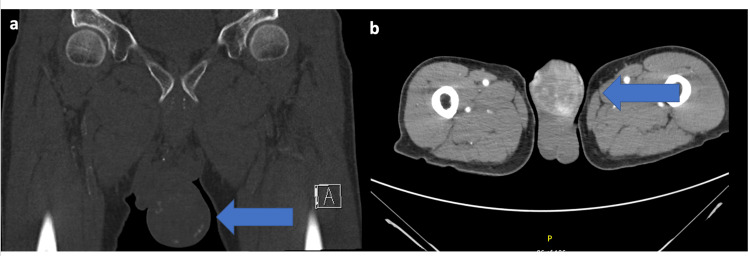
Computed tomography scan showing the heterogenous penile mass with areas of calcifications a. Coronal section; b. Axial section.
